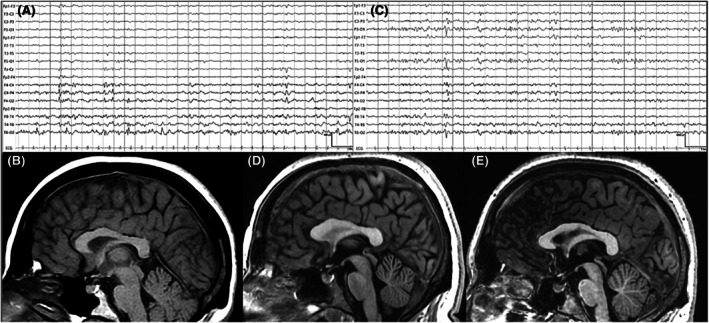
Electroencephalographic (EEG) and qualitative brain 3‐T magnetic resonance imaging (MRI) features. Interictal bipolar anterior–posterior EEG of the proband (A) and the proband's brother (C) showing prominent temporoparieto‐occipital interictal epileptiform discharges. Three‐dimensional T1‐weighted midsagittal brain MRI of the proband (B), the proband's brother (D), and the proband's sister (E) showing subtle dysmorphic features of the corpus callosum.
